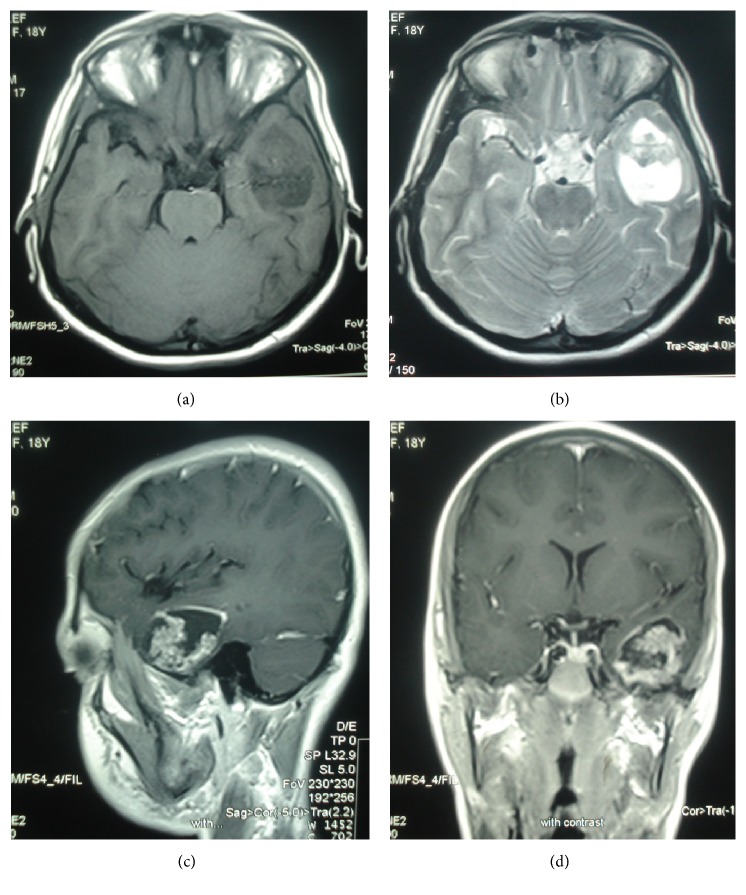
Craniopharyngioma of the left temporal lobe. Axial T1-weighted (a) and T2-weighted (b) MR images show a solid-cystic mass with no significant edema at left temporal lobe. Sagittal (c) and coronal contrast enhanced T1-weighted (d) MR images show peripheral wall enhancement and enhancing solid component.
